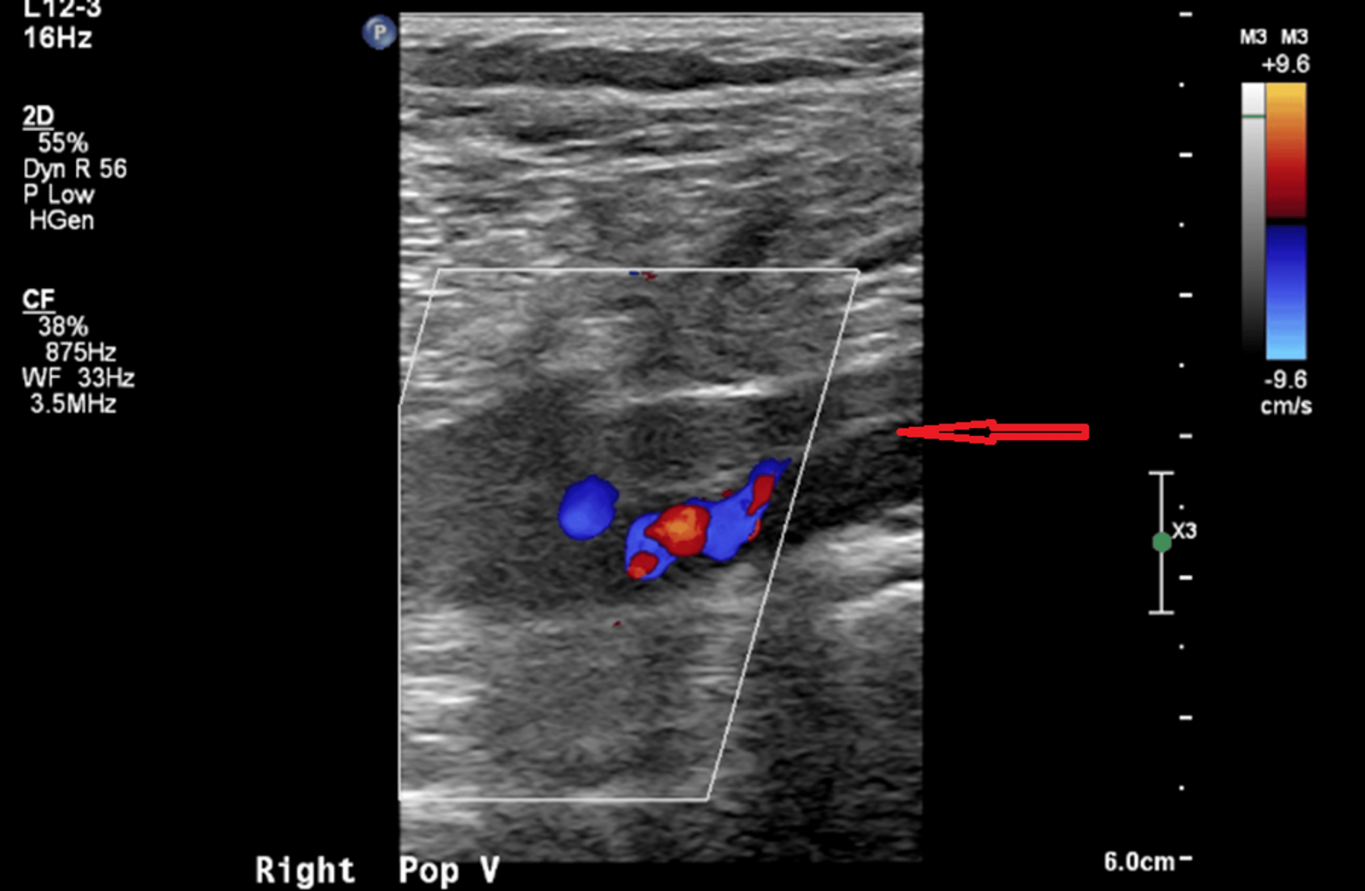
Ultrasound image of right popliteal vein thrombosis Compression ultrasound of the right popliteal fossa showing a non-compressible, dilated right popliteal vein (arrow), consistent with acute deep vein thrombosis.
